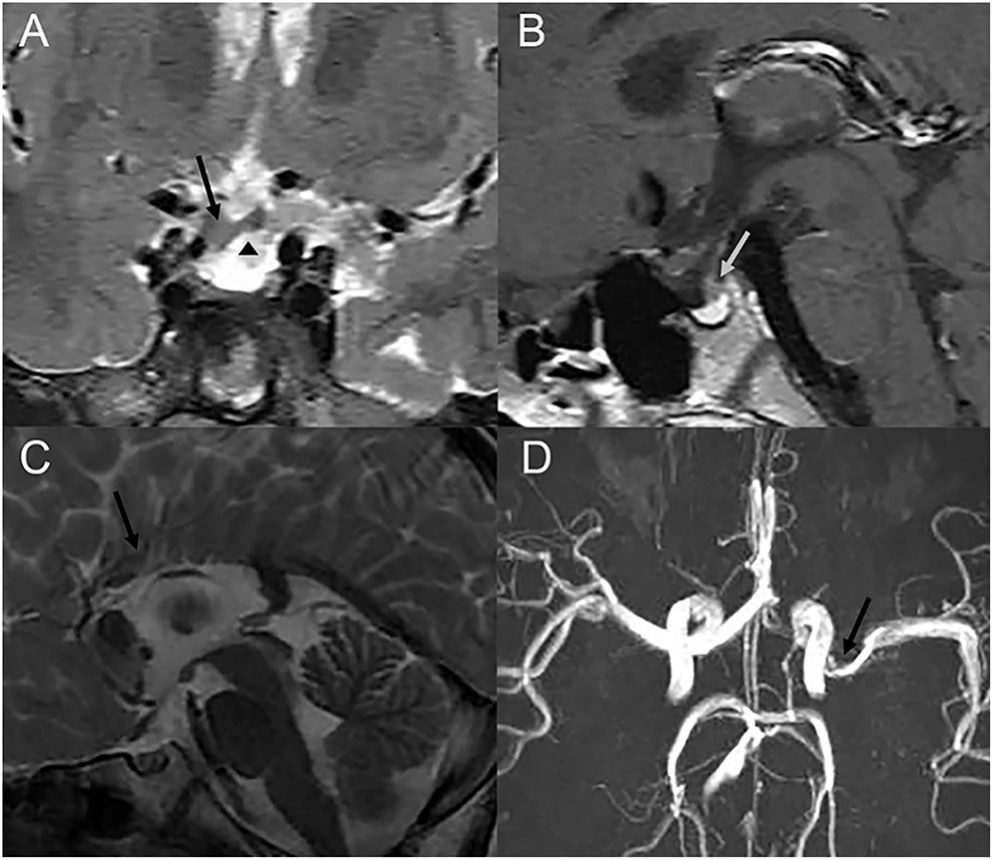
Cranial MRI or MRA images of three patients with cranial abnormalities. (A,B) Coronal T2-weighted and sagittal T1-weighted images of one patient, who showed growth hormone deficiency, showing a thickened optic chiasm (a black arrow), an ectopic pituitary (a triangle), and abnormal pituitary stalk (a grey arrow). (C) Sagittal T2-weighted images of one patient showing the corpus callosum body agenesis. (D) Cranial MRA showing the narrowing of the left M1 MCA segment (an arrow).
